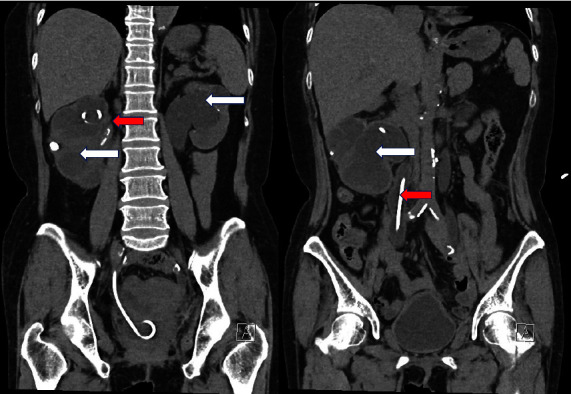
Urinary computed tomography scan showing bilateral ureterohydronephrosis (white arrow) with right double J stent (red arrows).
